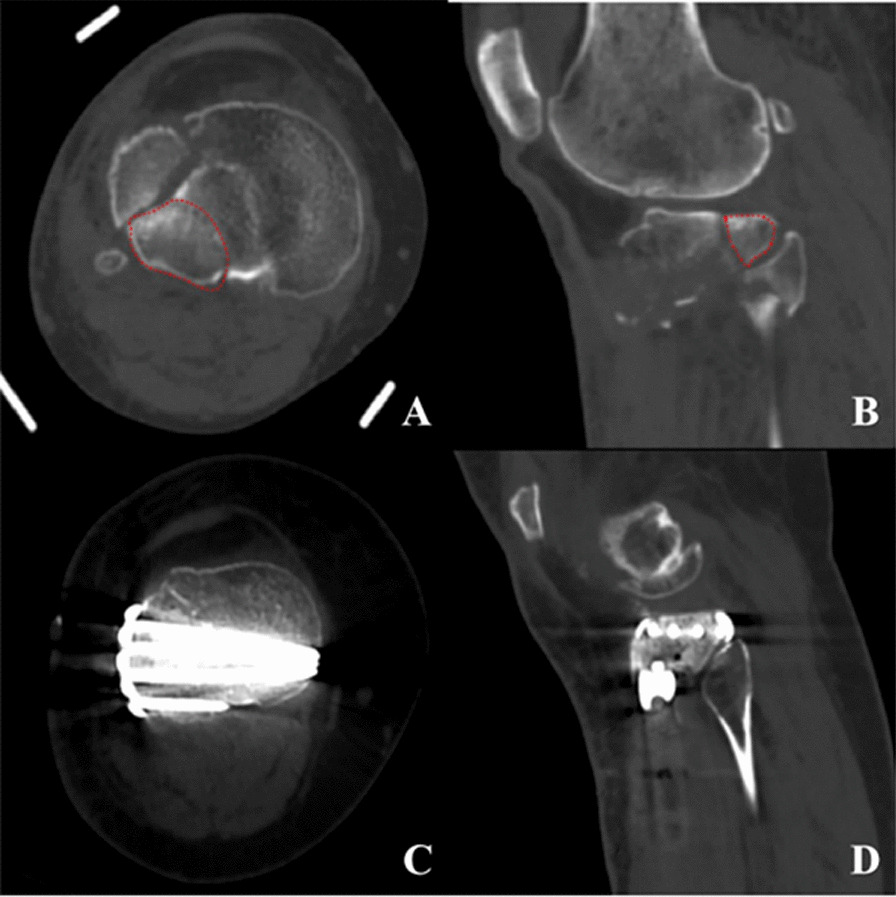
CT Image of Schatzker type II tibial plateau fracture involving the posterolateral plateau. A Preoperative transverse image, B preoperative sagittal image, C postoperative transverse view showing plate posterior placement up to two screw fixation, D postoperative sagittal view showing posterior placement of the plate up to two screws for fixation
